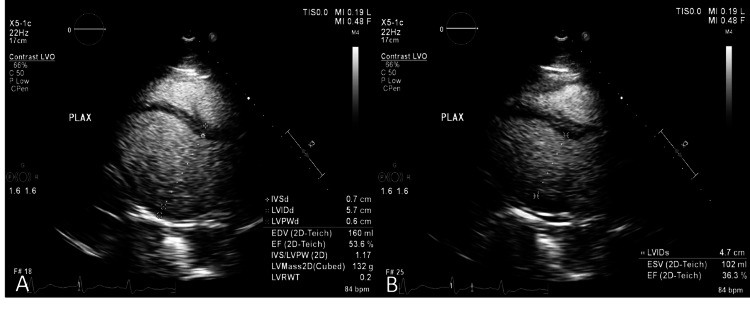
TTE parasternal long-axis view at end-diastole (A) and end-systole (B) demonstrating a reduced ejection fraction (patient had poor apical windows) TTE: transthoracic echocardiogram
